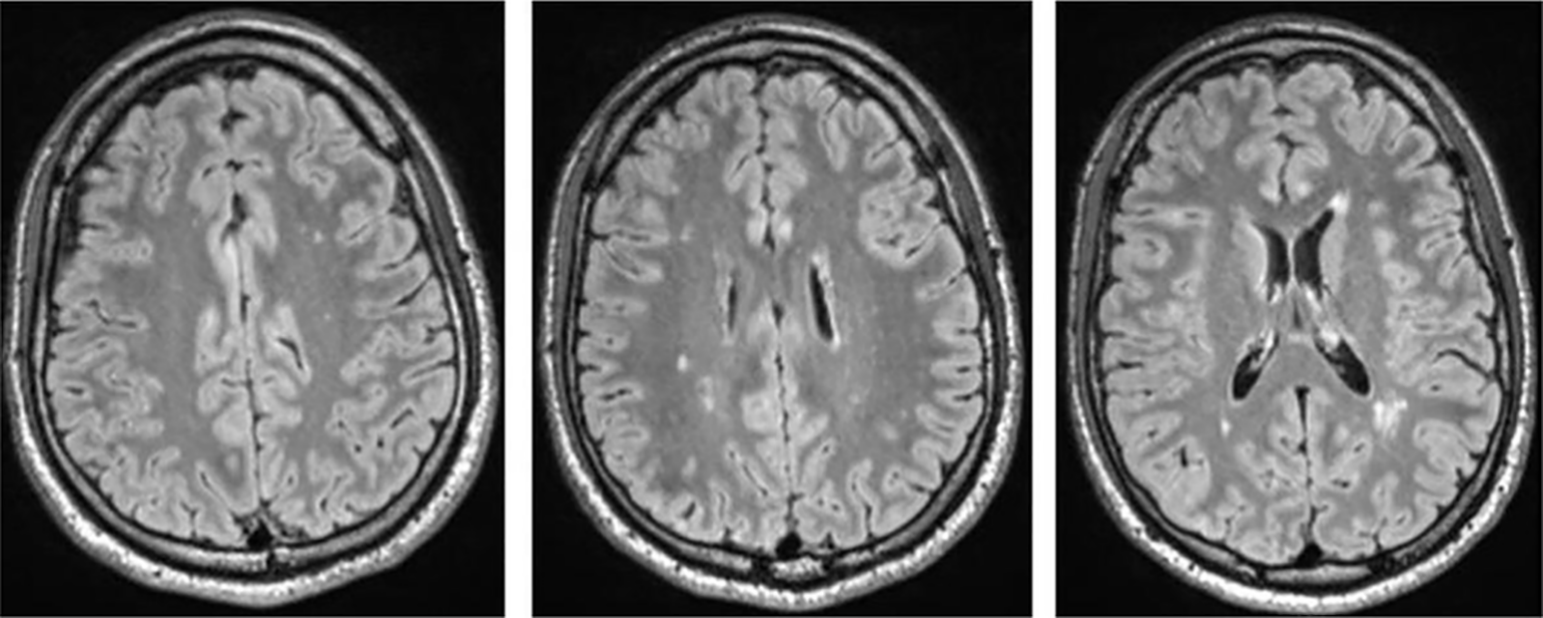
T2w fluid-attenuated inversion recovery (FLAIR) cerebral magnetic resonance imaging (cMRI) shows multiple disseminated dotted bilateral periventricular to subcortical white matter lesions. Shown are images of the first cMRI performed 6 months after symptom exacerbation in November 2016.
